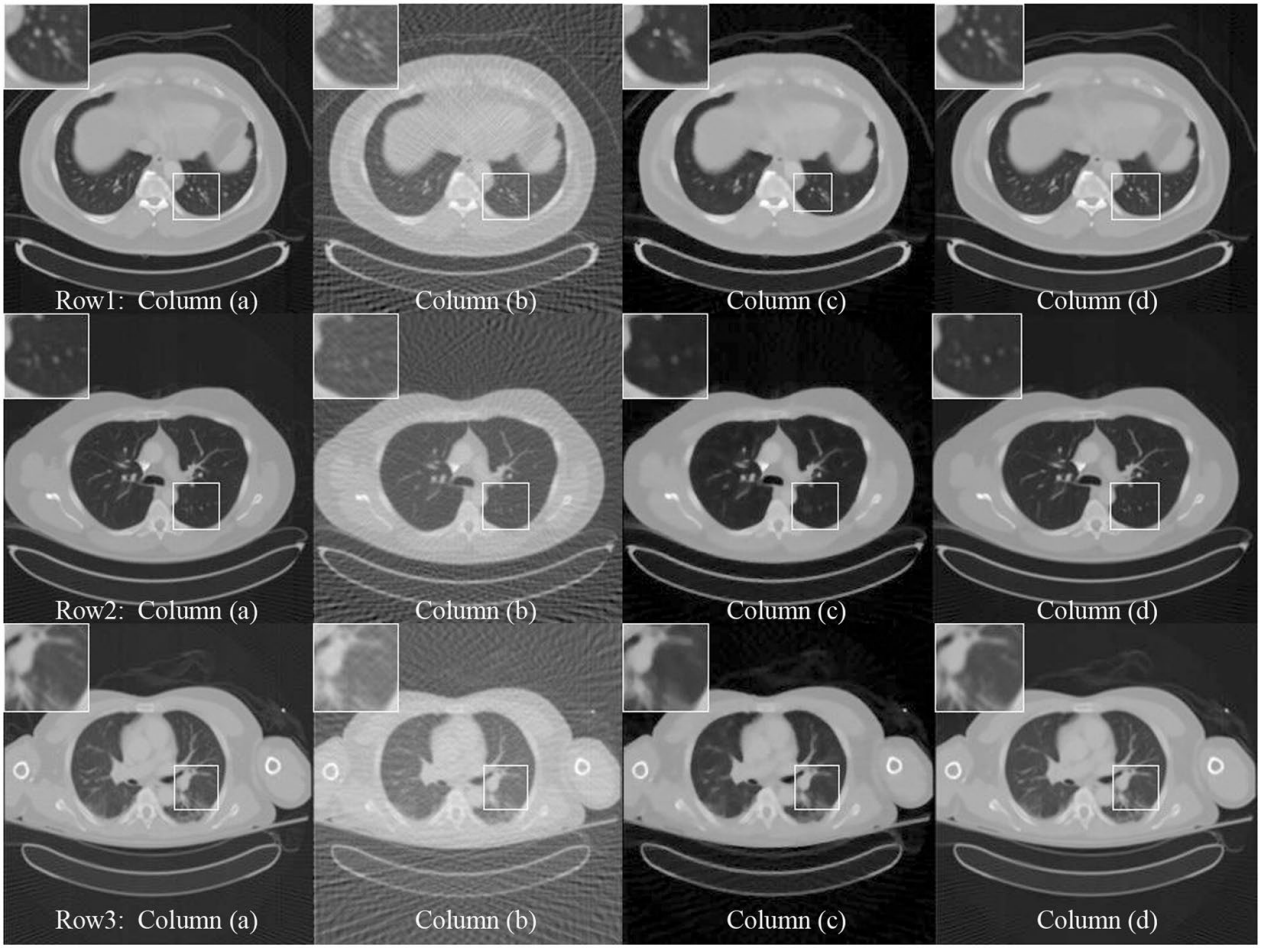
The 256*256 CT images reconstructed by 120 projection views. Column (a) shows the reconstruction by the FBP method from the full projection views. Column (b) shows the reconstruction by the FBP method from the sparse projection views. Column (c) shows the reconstruction by the ADS-POCS method from the sparse projection views. Column (d) shows the reconstruction by the proposed method.
